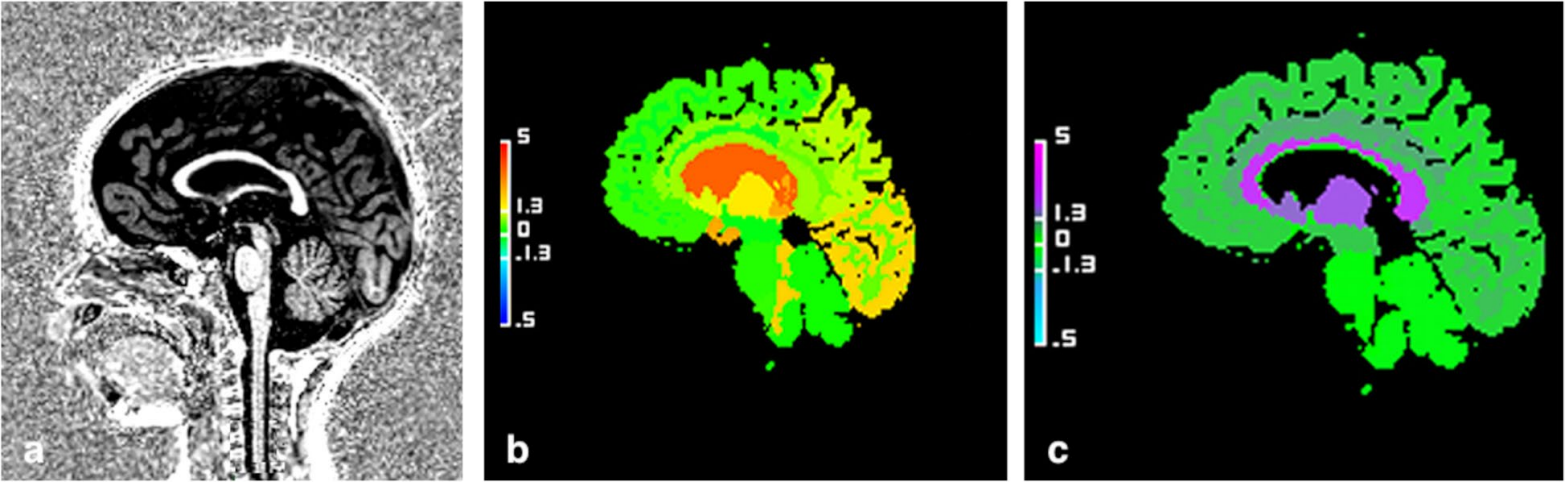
Example of a 2-year-old and 7-month-old child with neurodevelopmental disorders with an isolated T1 relaxometry anomaly. Midline sagittal reconstructions. a High-contrast uniform T1-weighted anatomical sequence showing a corpus callosum of normal shape and thickness. b Map of normal volumetric deviations, with CC appearing in green, *Z*-score = 0.49. c Map of abnormally increased T1 relaxometric deviations, with CC appearing purple, *Z*-score = 2.20
